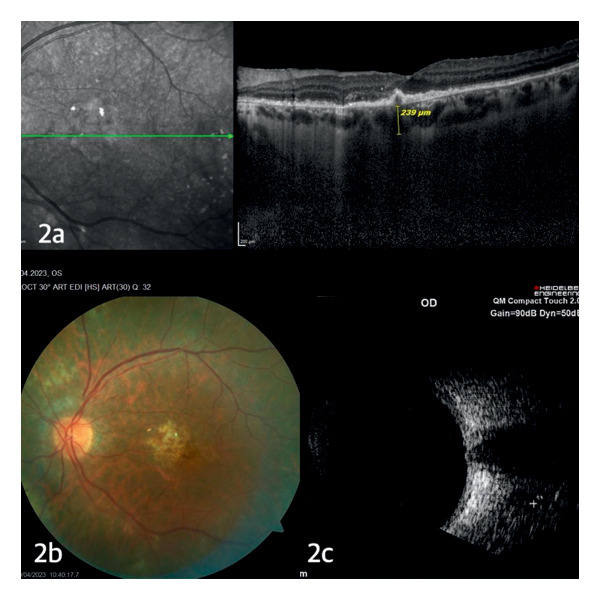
(a–c) Fundoscopic, OCT, and ultrasonographic evaluation after the fourth dose of high‐dose methotrexate treatment showed significant reduction of the choroidal infiltration.
